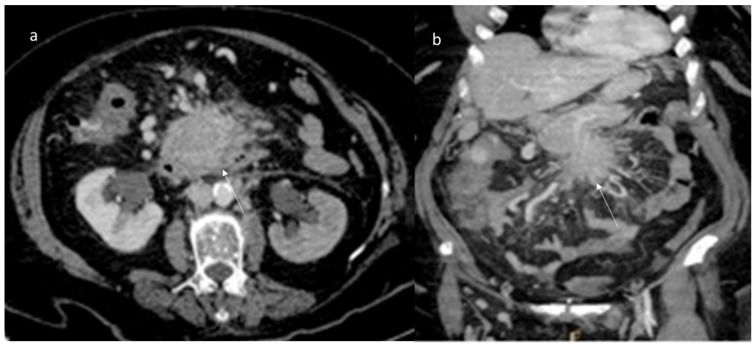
Axial (a) and MPR coronal (b) CT assessment of aggressive fibromatosis (arrow) in portal phase of contrast study. The lesion shows mild enhancement and involvement of blood vessels.
